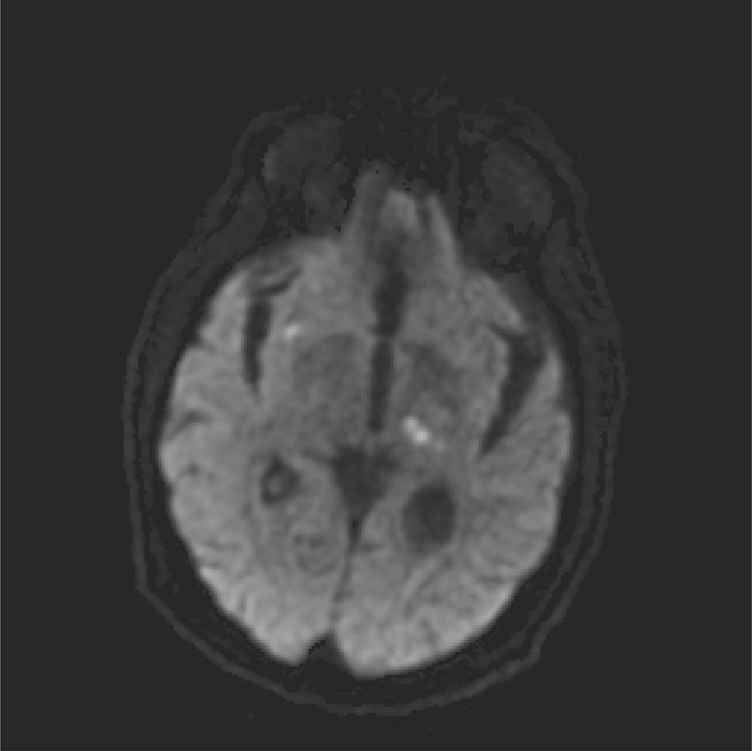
MRI demonstrating bilateral infarcts.
